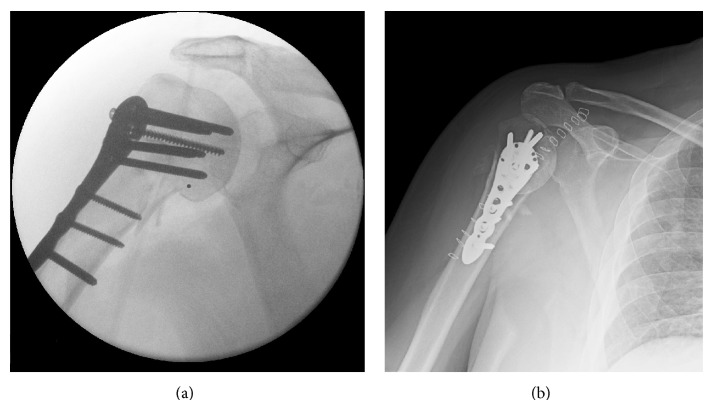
Radiograph showing the proximal humerus after reconstruction with a plate and screws (a) during surgery and (b) 14 days later when the screws had pulled out from the humeral head.
